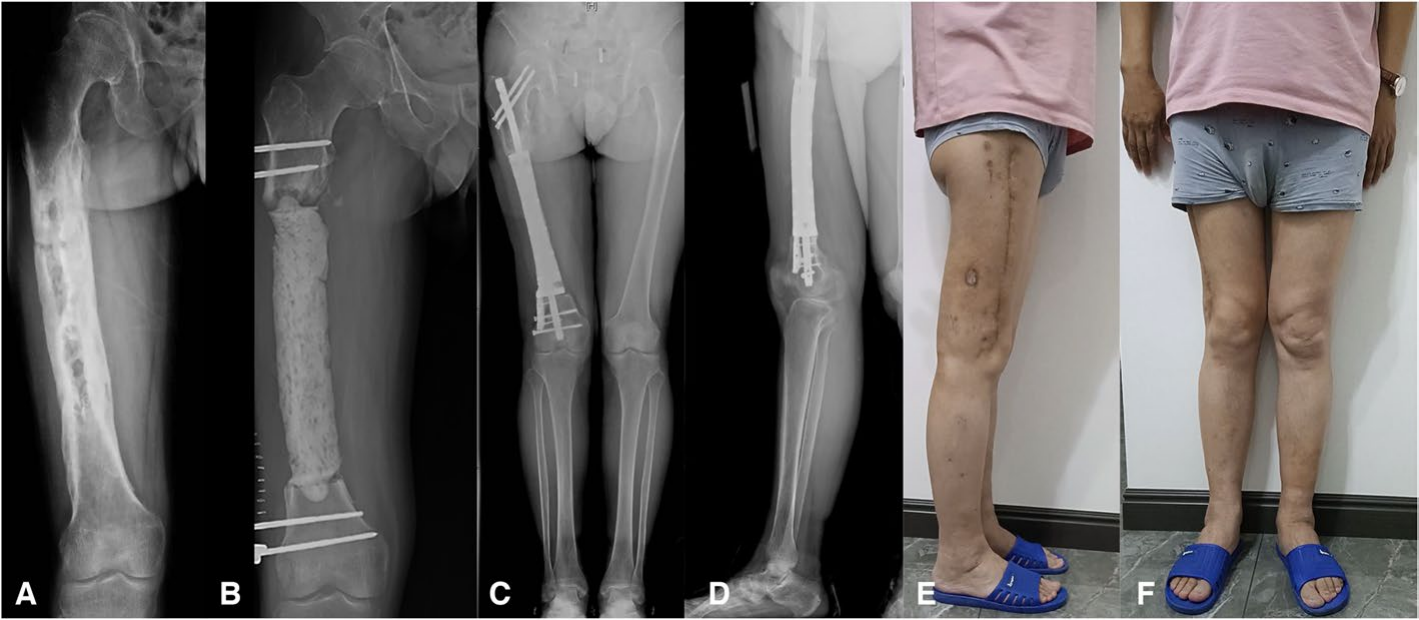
A A 51-year-old patient suffered osteomyelitis after a femur injury. B After debridement three times, an external fixator was applied, and PMMA was used to fill the spacer. The length of defect was 26.1 cm. C and D Anteroposterior (C) and lateral radiographs (D) showing the stability of the reconstruction system after 2 years. The bone growth could be observed proximally. E and F There was no limb-length discrepancy. The patient achieved full weight-bearing and was able to resume his original work. PMMA = polymethylmethacrylate
